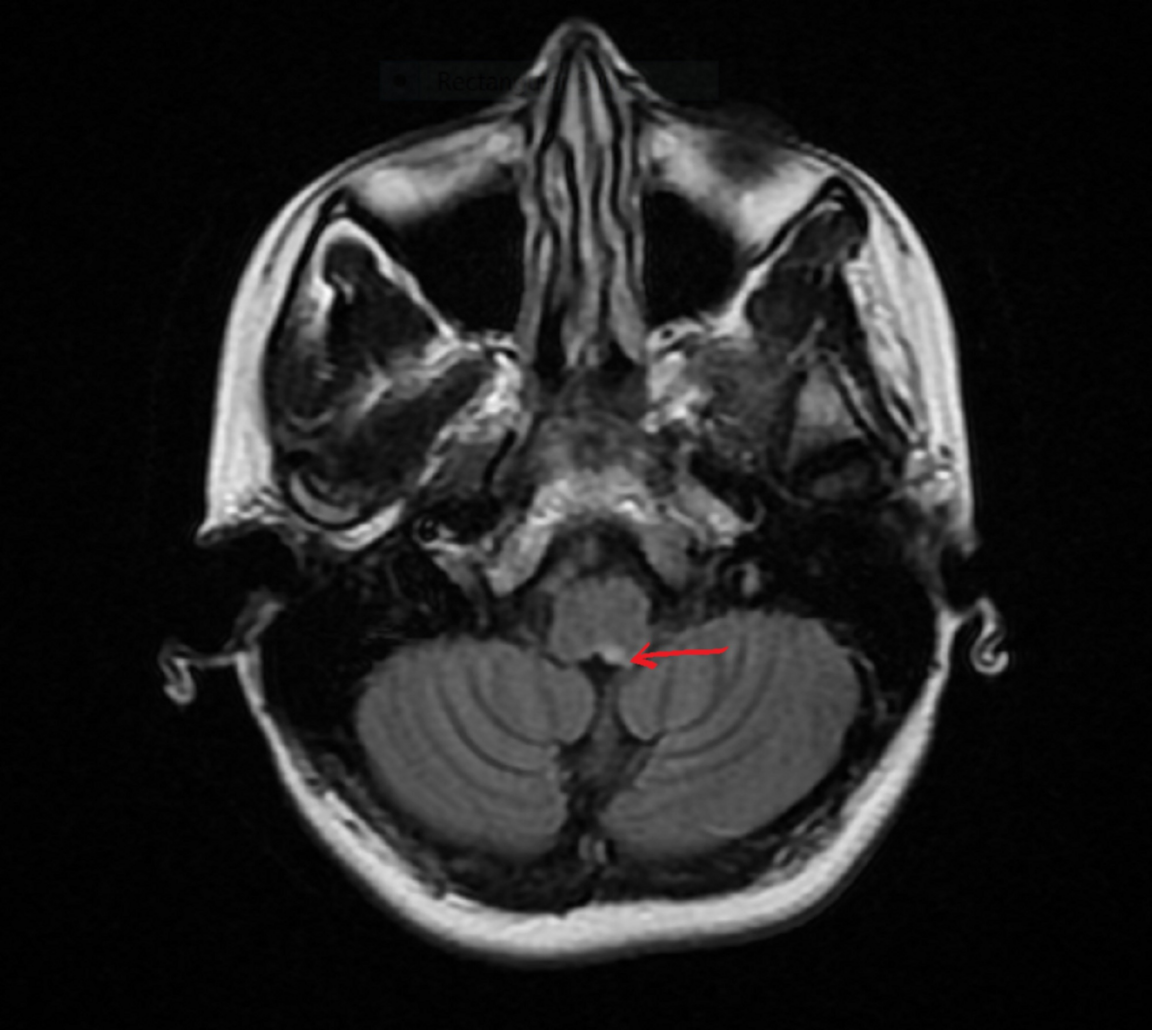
MRI brain FLAIR sequence showing demyelinating lesion in left medulla-area postrema (arrow pointing).
